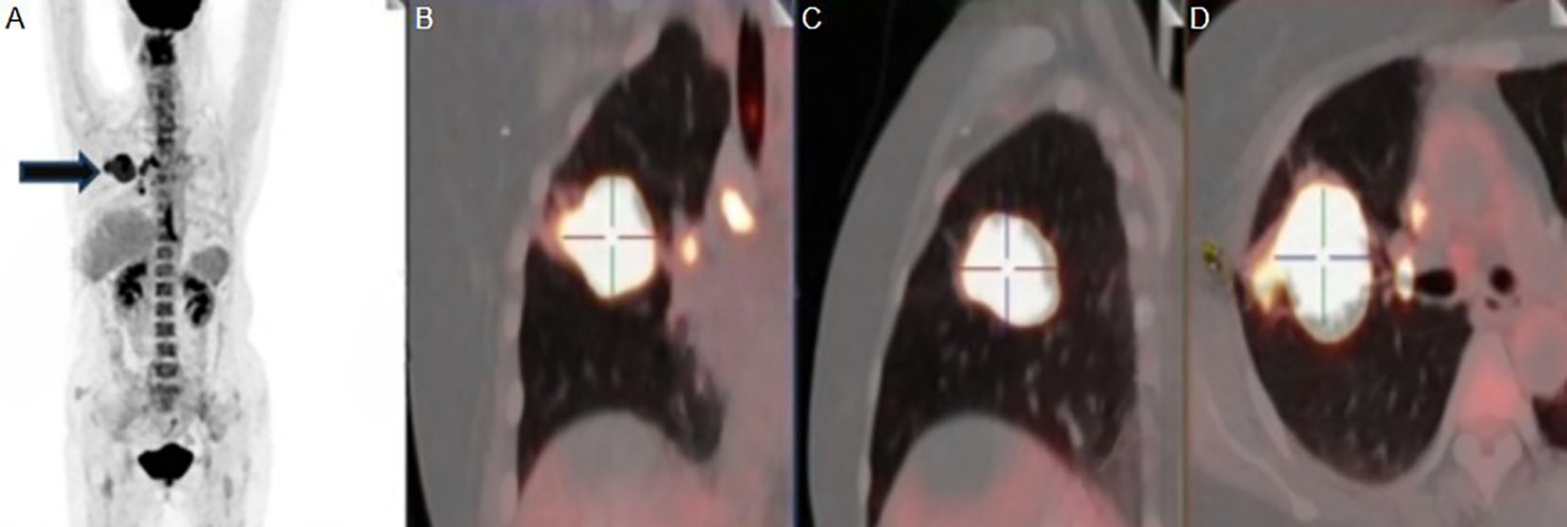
(A) MIP image in Positron emission tomography-computed tomography (PET-CT) of the patient and the black arrow points to the right lung lesion. (B, C) A solid mass in the upper lobe of the right lung with abnormal metabolism. (D) The apical and posterior segments of the right upper lobe are truncated by the tumor. MIP, Maximum Intensity Projection.
